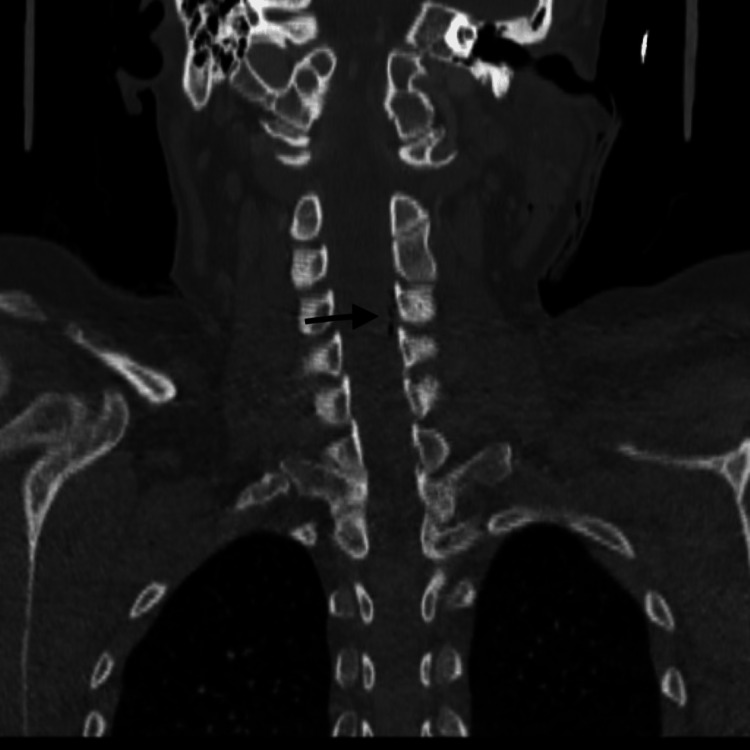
CT image showing multiple extradural air foci on the left cervical spinal from C4 to C6 level
